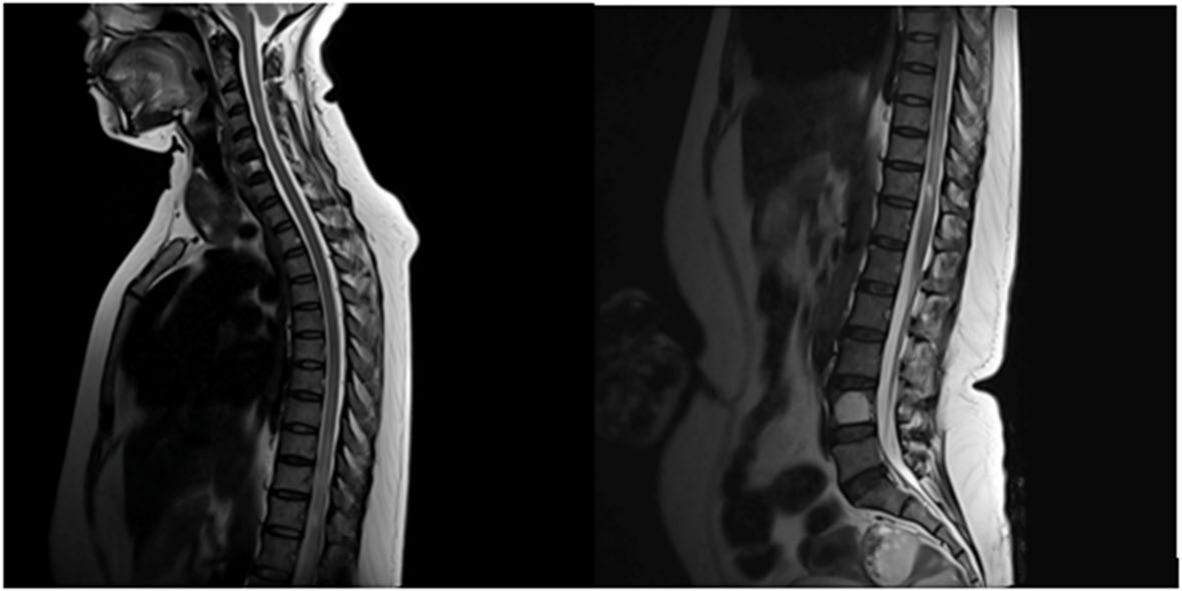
T1 fat saturated contrast MRI spine shows contrast enhancement of the whole spinal cord
